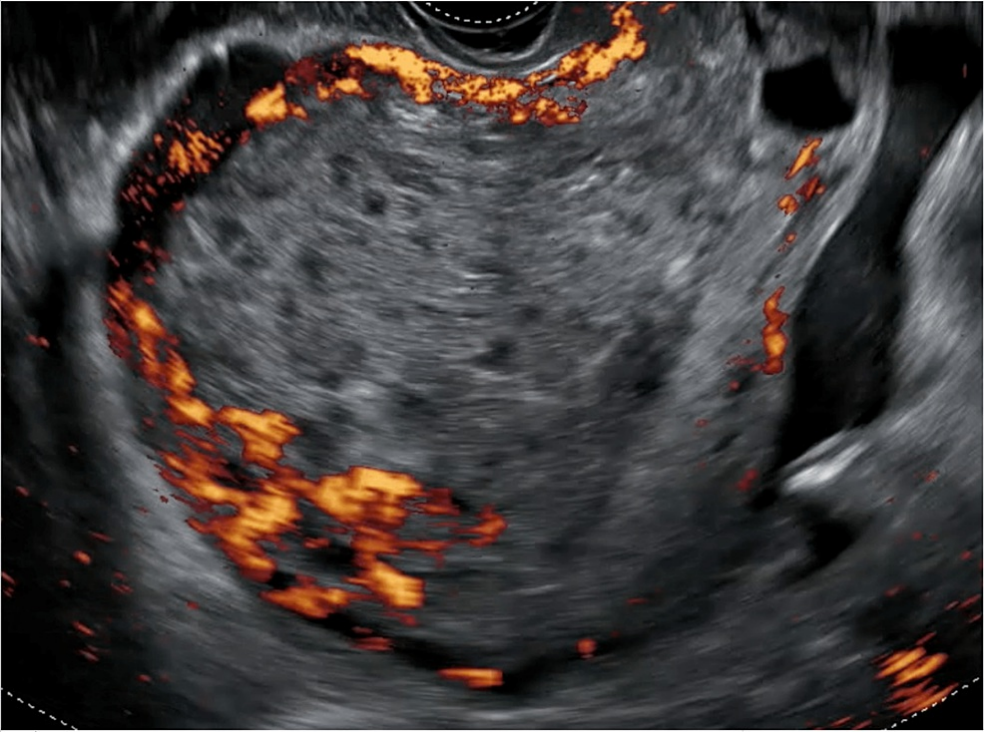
Color Doppler ultrasonography exhibits high vascularization of the endometrial lesion
